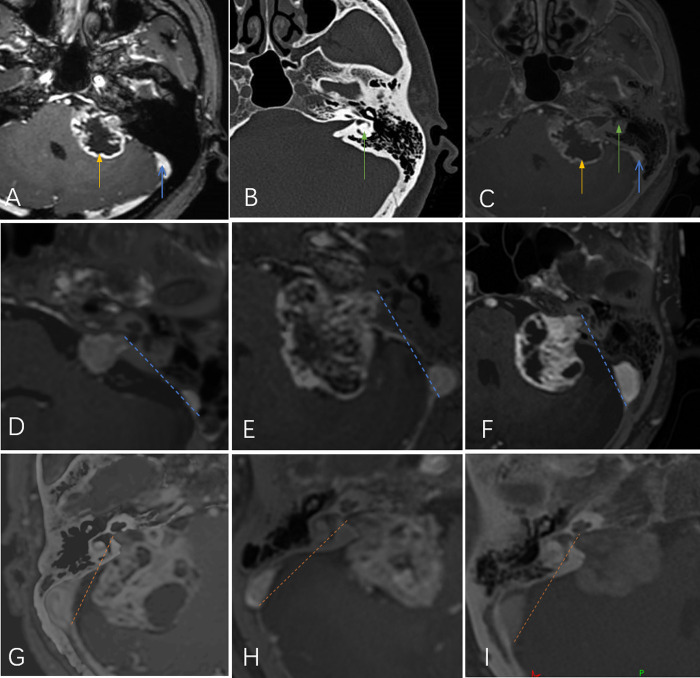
(A) Magnetic resonance imaging shows the tumor (yellow arrow) and sigmoid sinus (blue arrow). (B) Computed tomography shows the labyrinth (green arrow). (C) The fused image shows the positions of all three simultaneously. The line between the outermost edge of the tumor and the medial side of the sigmoid sinus was defined as the lateral safe line (LSL; yellow- or blue-dotted line). Tumors were classified according to the relationship between the LSL and the labyrinth: lateral type (D,E,F) and medial type (G,H,I).
